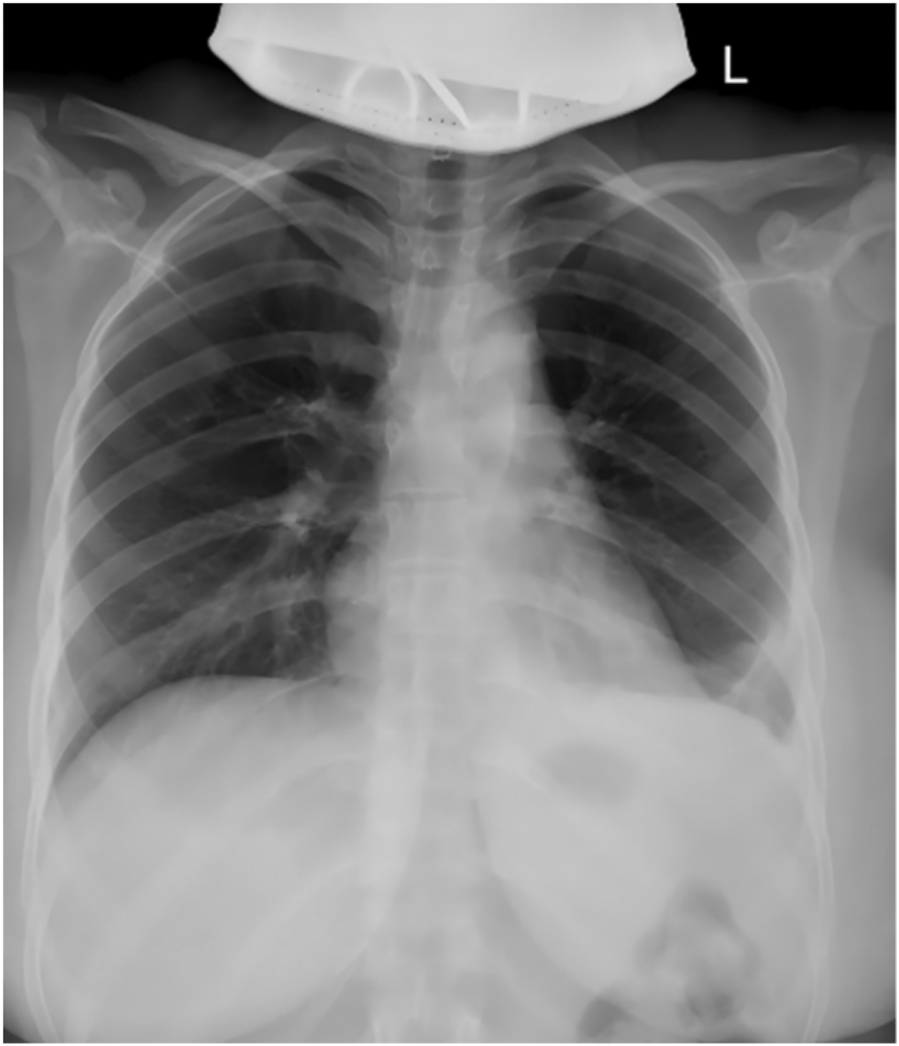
Postoperative follow-up chest x-ray, indicating complete removal of the lesion.
